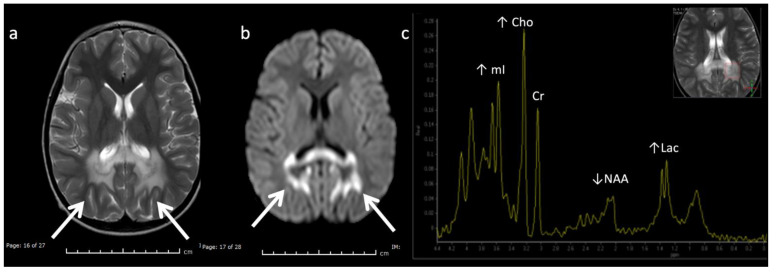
Six-year-old male with X-linked Adrenoleukodystrophy (ALD). (a) Axial T2 and (b) DWI images reveal confluent T2 hyperintensity (a) and reduced diffusion (b) involving the callosal splenium and forceps major/peri-trigonal white matter (white arrows) with sparing of subcortical U-fibers. The pattern of involvement has a postero-anterior and centrifugal pattern. A zonal pattern of signal alteration with mixed diffusion abnormalities indicates acute on chronic demyelination related injury. (c) SV-MRS of the left periatrial white matter reveals decreased NAA (neuronal loss, decreased neuronal-axonal integrity, and/or decreased production), elevated Cho (increased membrane turnover), increased mI (neuroinflammation marker), and increased Lac (anaerobic metabolism).
